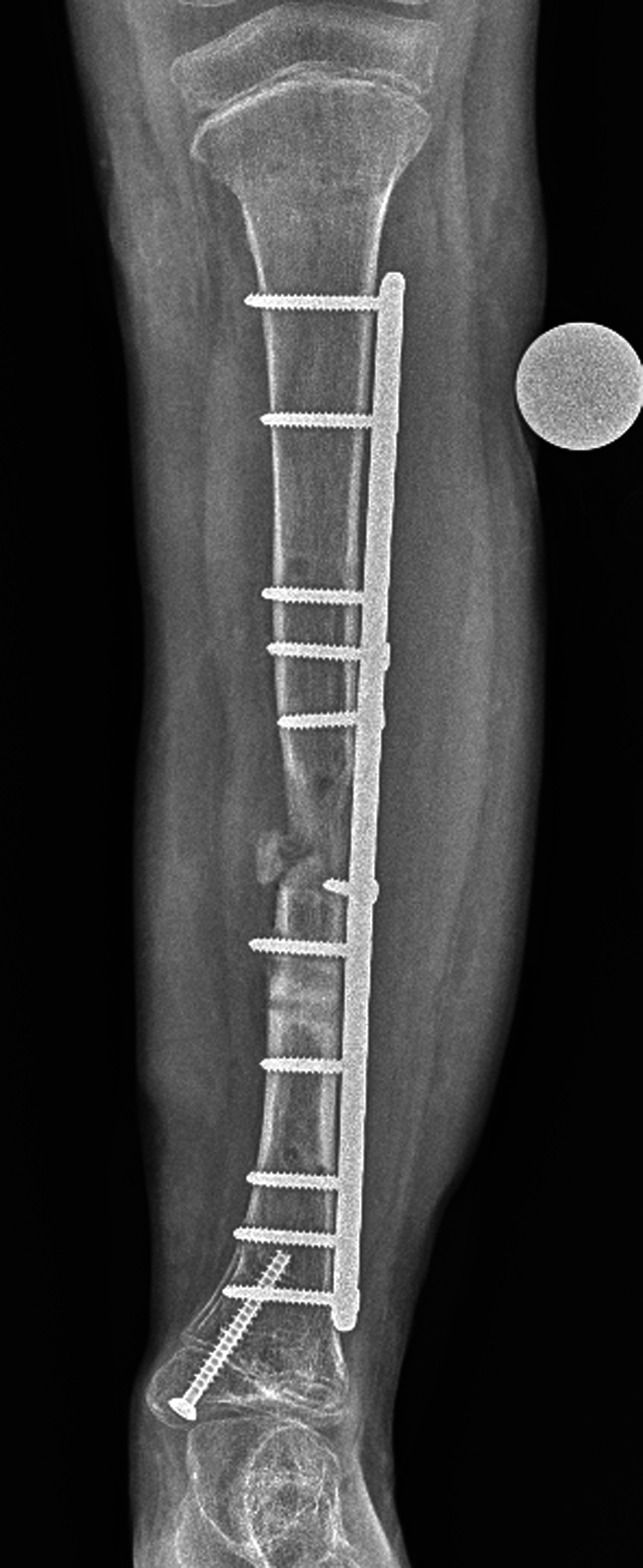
Postoperative AP radiograph demonstrating a left tibia after an external fixation-assisted plate exchange for tibial realignment.
